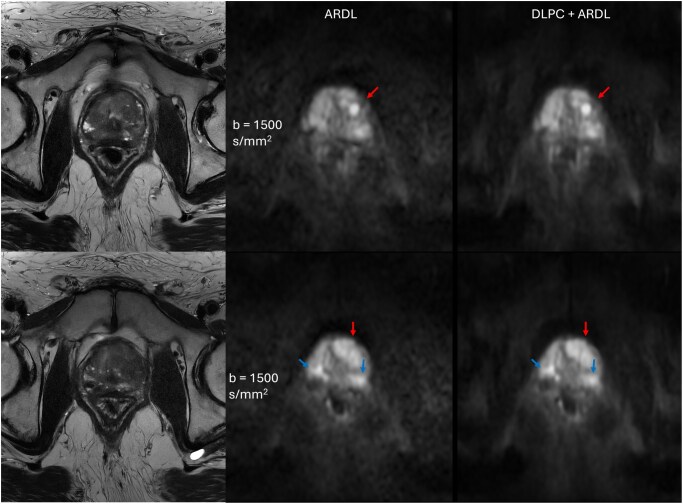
Patient images demonstrating improved small detail visibility in diffusion weighted images (DWI) reconstructed with DL Phase Correction with ARDL (DLPC + ARDL, right) in comparison to product AIR Recon DL (ARDL, middle) for detection of multifocal lesions and extraprostatic extension in the base (top) and midgland (bottom). Anatomical references are shown in the T2-weighted (T2w) images (left). This patient is 61 years old with a prostate-specific antigen  = 14.8 ng/mL, with a large transition zone cancer (Gleason 4 + 3) that almost extends to the capsule (red arrow) and was pathologically confirmed with the presence of extraprostatic extension along with left and right peripheral zone cancers (blue arrows). The left peripheral zone Gleason 4 + 3 cancer touching the left neurovascular bundle had a pathology-confirmed presence of perineural invasion. With DLPC + ARDL reconstruction, the reduced noise levels improve visibility of smaller structural details within the right peripheral zone (Gleason 4 + 5) lesion.
